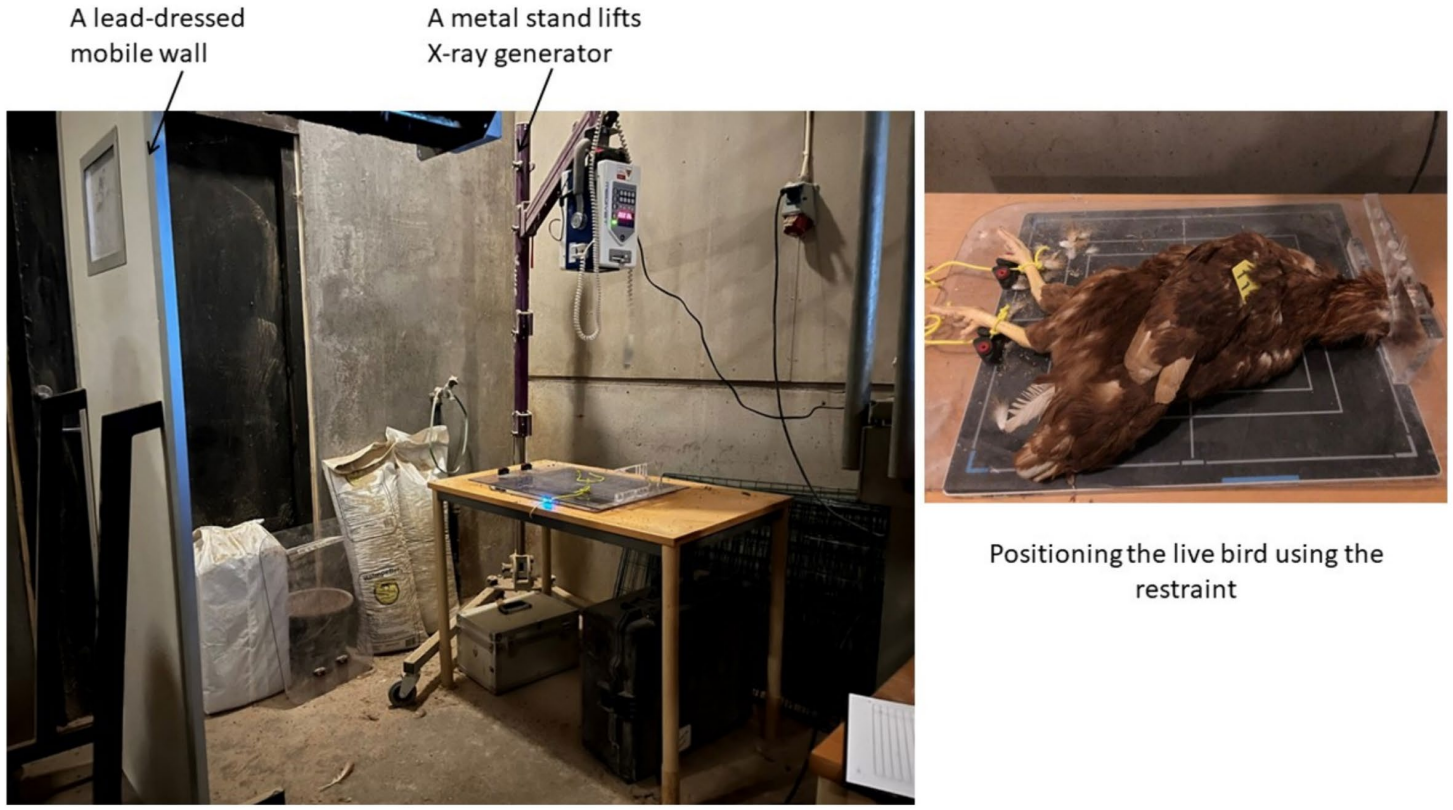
(Left) Set-up used for on-farm radiographic examination and (right) a live bird restrained and positioned for radiographic examination. The distance between radiography source and the flat panel detector was 100 cm.
